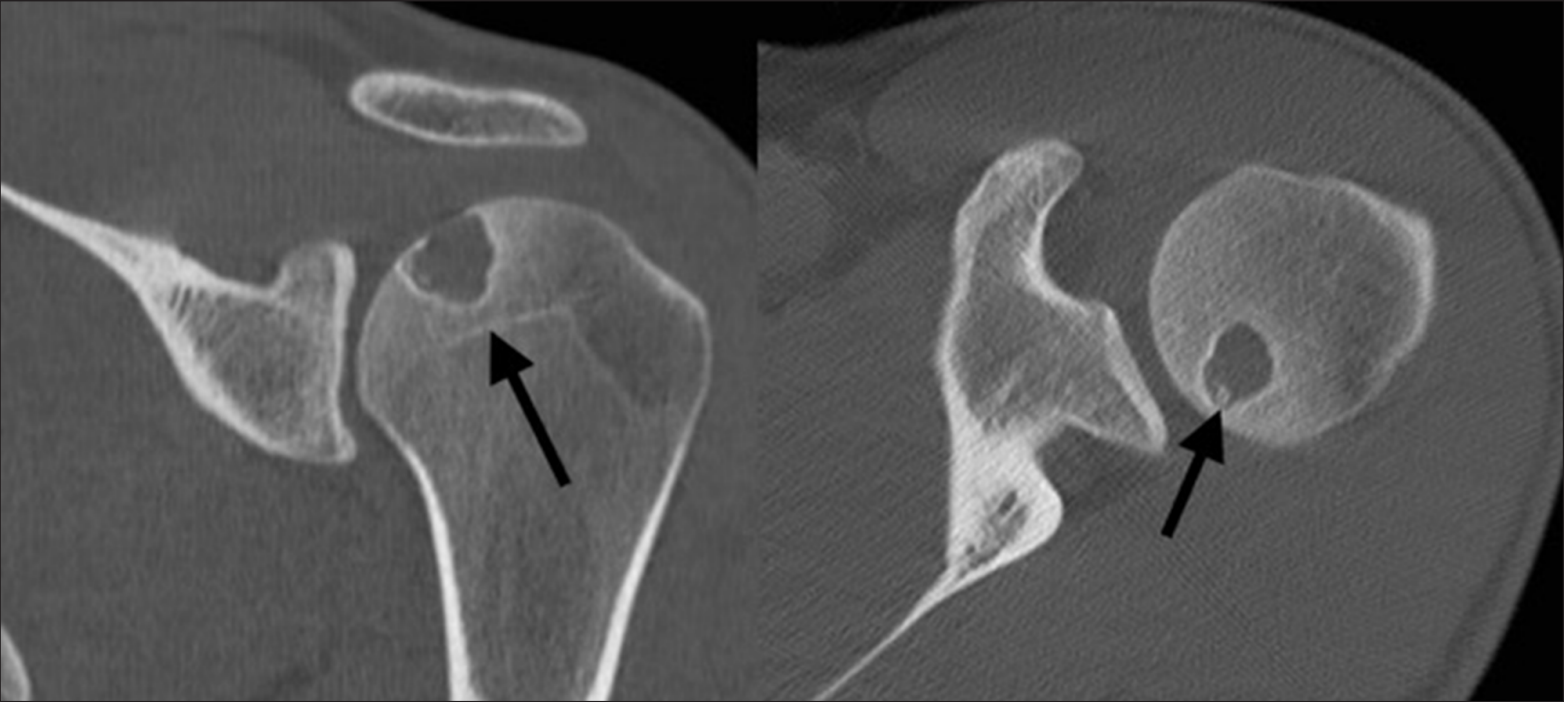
CT of the left shoulder. (A) Coronal reformatted image: the lesion does not reach the growth plate, which is already closed (arrow); (B) Axial plane image: a few small dense fragments can be seen in the lytic lesion (arrow): calcifications, confirming the presence of a chondroid matrix.
